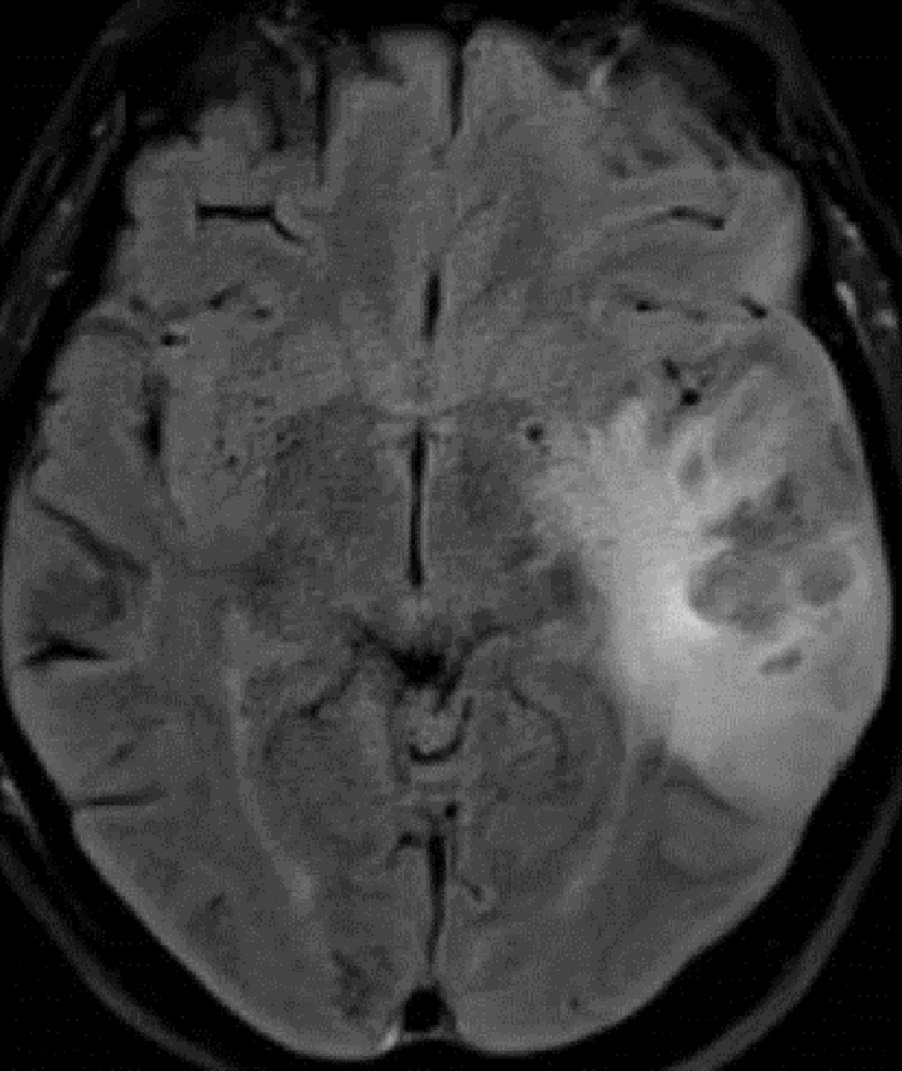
T2 MRI at Presentation T2 MRI in the axial plane obtained at presentation demonstrates classic venous infarct in the left temporal lobe.
